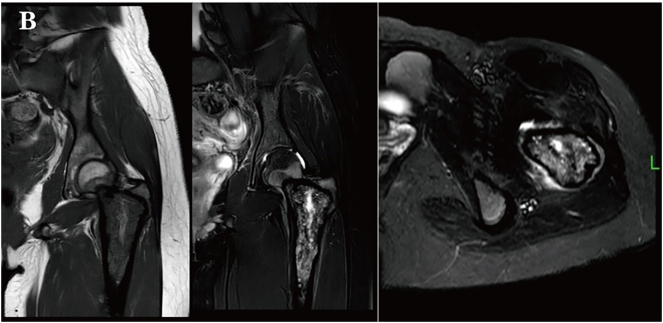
MRI demonstrated a mixed long T1 and long T2 signal in the proximal left femur.
